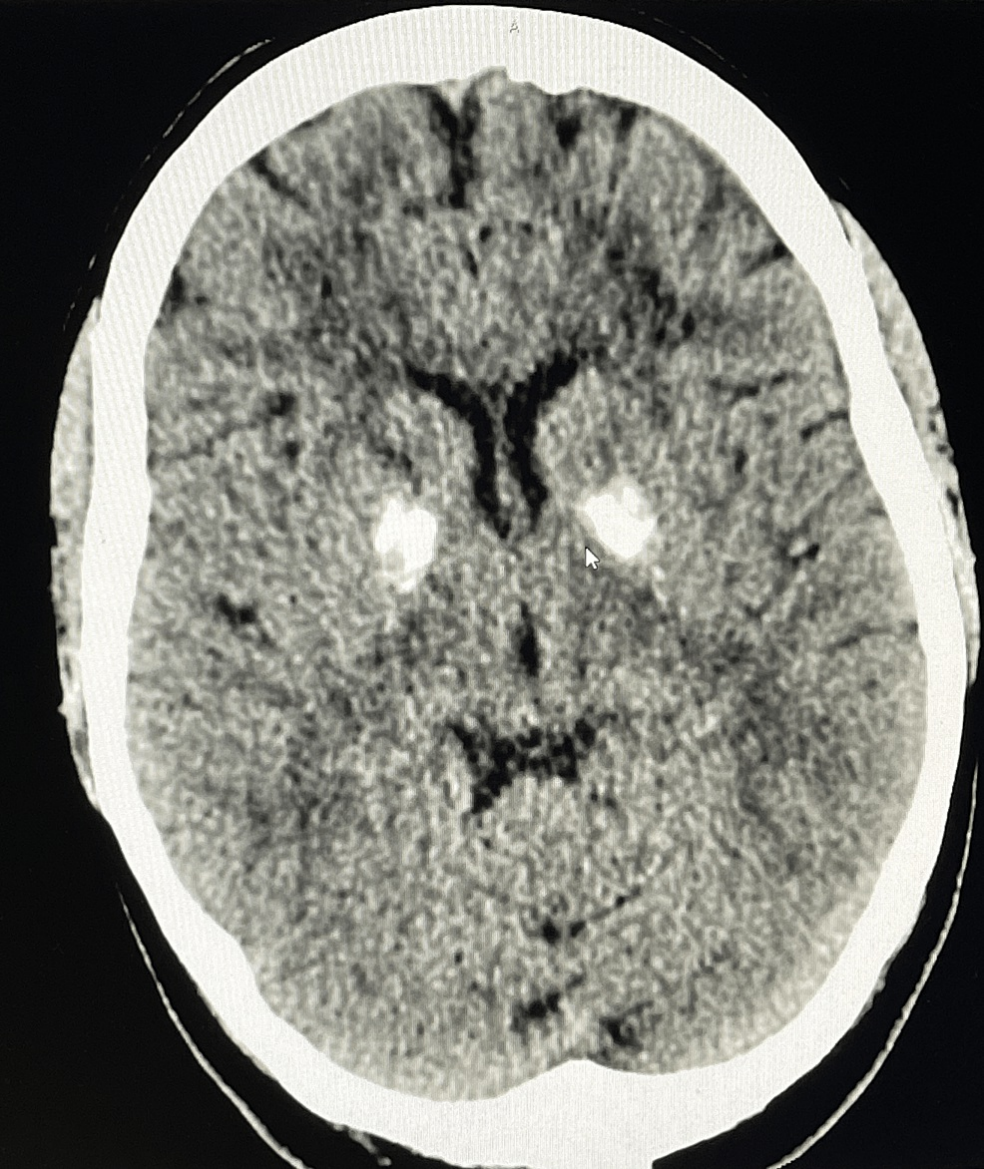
CT scan of the head showing bilateral basal ganglia calcification
